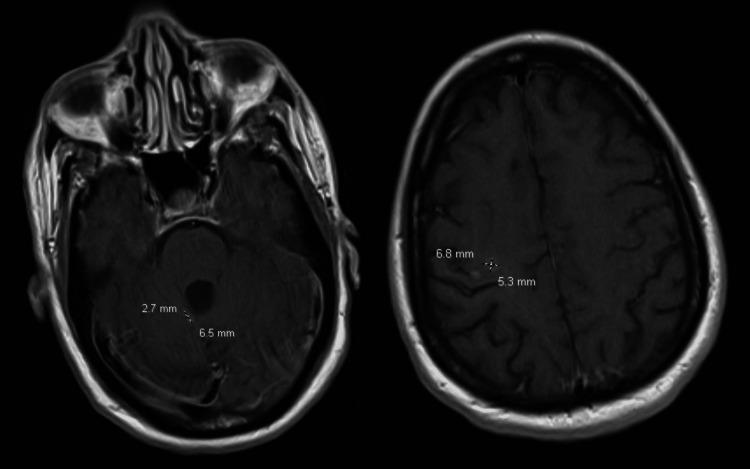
Axial T1 brain MRI images at one year post-admission The image on the left shows the right paravermian cerebellar lesion, and the image on the right shows the posterior right frontal lobe lesion MRI: magnetic resonance imaging
